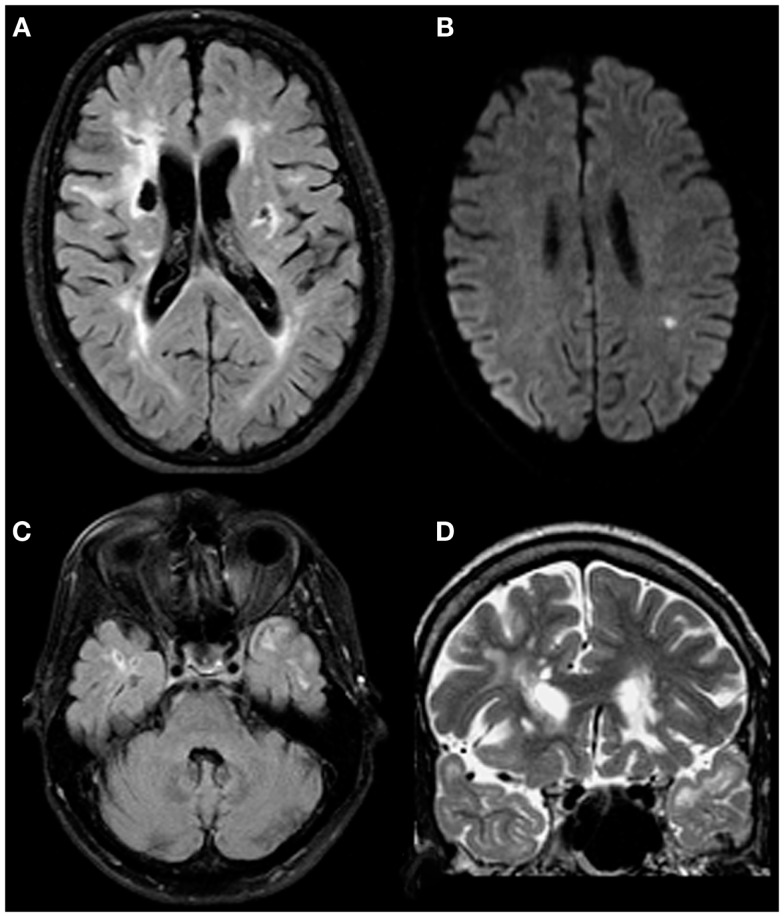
Both chronic and acute changes in a patient with proven CADASIL. Chronic lacunar defects and gliotic changes affecting bilateral fronto-parietal regions including basal ganglia on both sides [(A); axial FLAIR image]. Subacute lacunar infarct in the left parietal white matter [(B); axial diffusion weighted image]. Gliotic changes affecting both anterior temporal lobes are thought to be typical for CADASIL [(C,D); axial FLAIR and coronal T2 weighted images; please note: lacunar defects in the right temporal lobe are adjacent to the right-sided amygdala].
